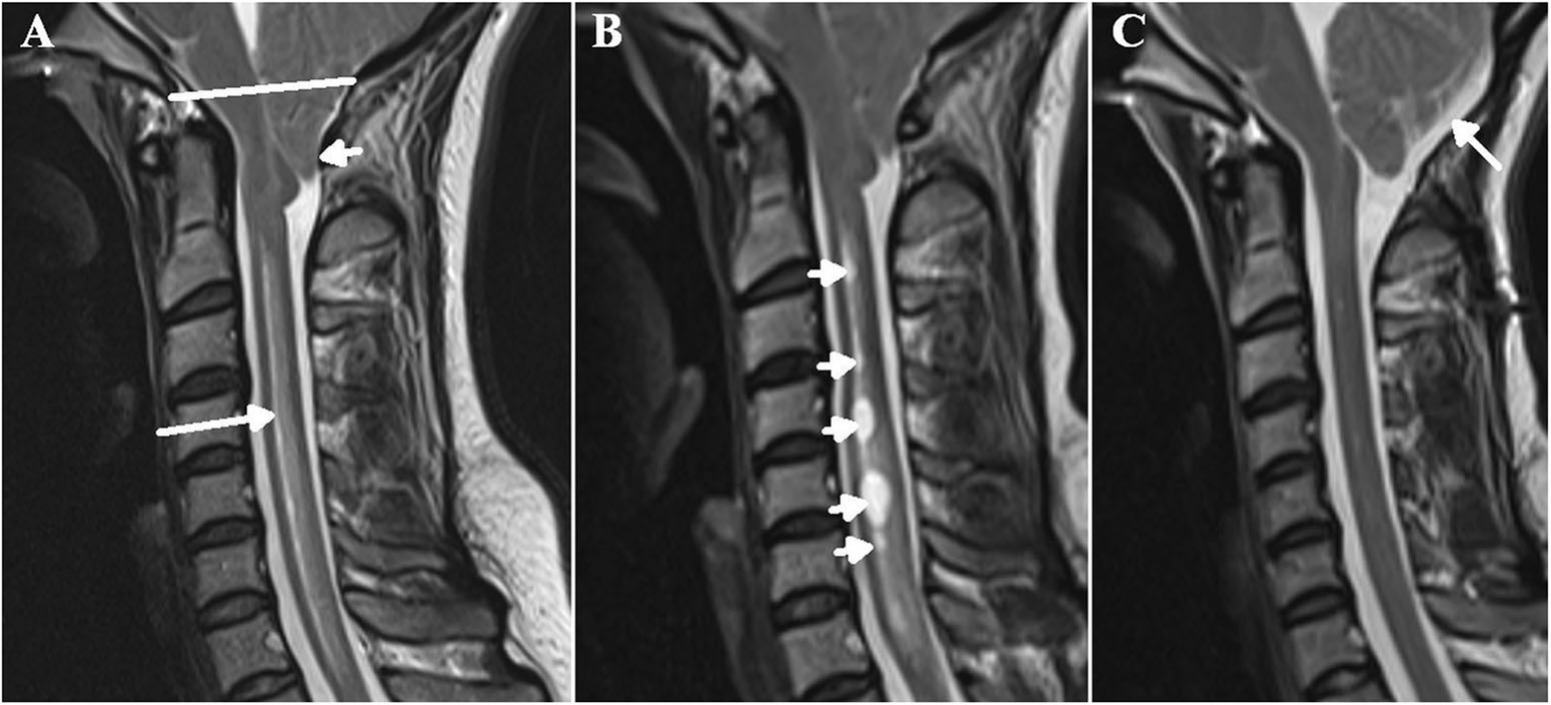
The progression of edema in syringomyelia. A T2 weighted sagittal MRI of the neck in a 28-year-old female patient who presented with headache on bending and coughing. There is a Chiari 1 malformation with significant cerebellar tonsil herniation (small white arrow) below the foramen magnum (white line) leading to reduced spinal canal compliance. There is spinal cord edema from C2 to T1 (long arrow) (A). One year later, following conservative management, there were multiple cystic cavities developing (arrows) (B). Following posterior fossa decompression (arrow), there is now improved compliance in the spinal canal with the resolution of the edema and the cystic cavities previously noted (C). Reproduced from Bateman and Bateman^15^ under a Creative Commons Attribution (CC BY) license.
